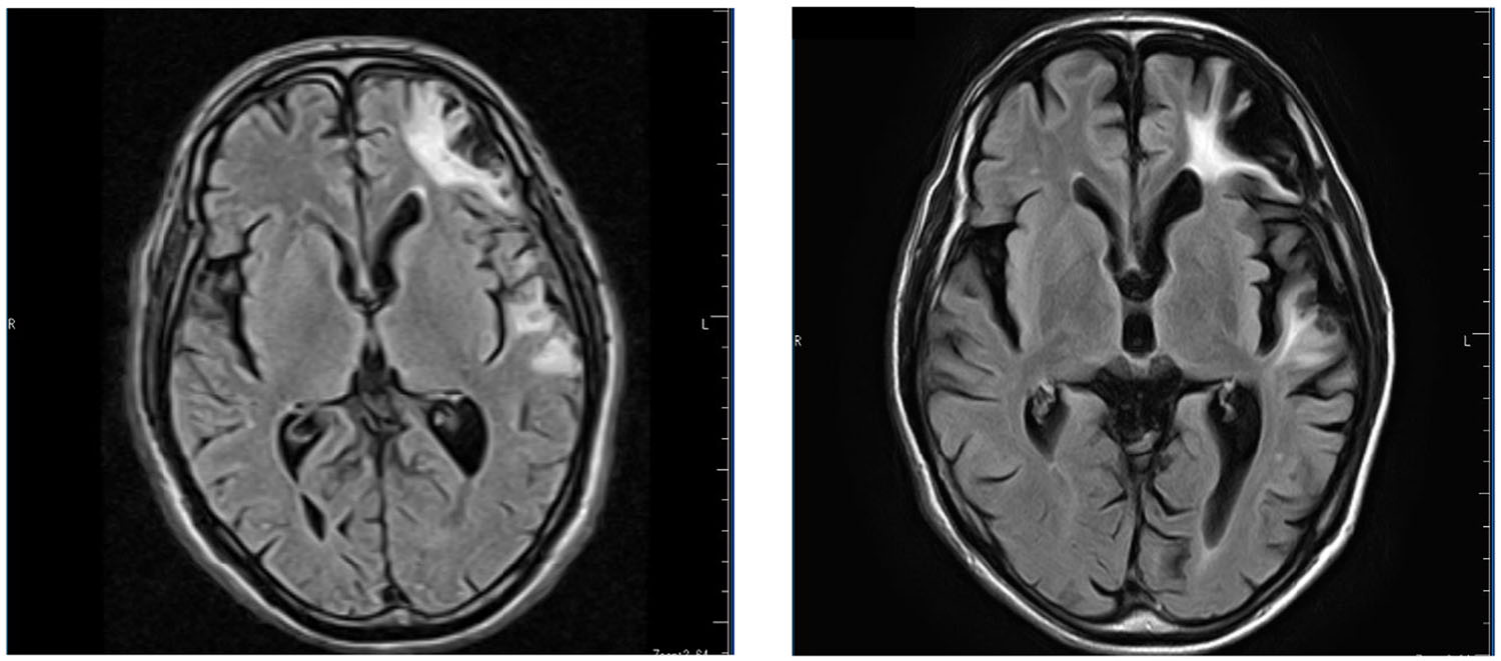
MRI FLAIR images of the patient before and after hospitalization. The left panel shows images at 1 year after the injury, and the right panel shows images on the day before hospitalization.
